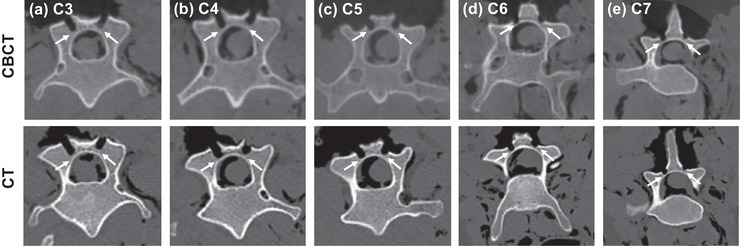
Postoperative CBCT (top) and CT (bottom) images of the cervical vertebra (C3–C7 (a)–(e), axial view) following the procedure. White arrows indicate the remaining lamina
